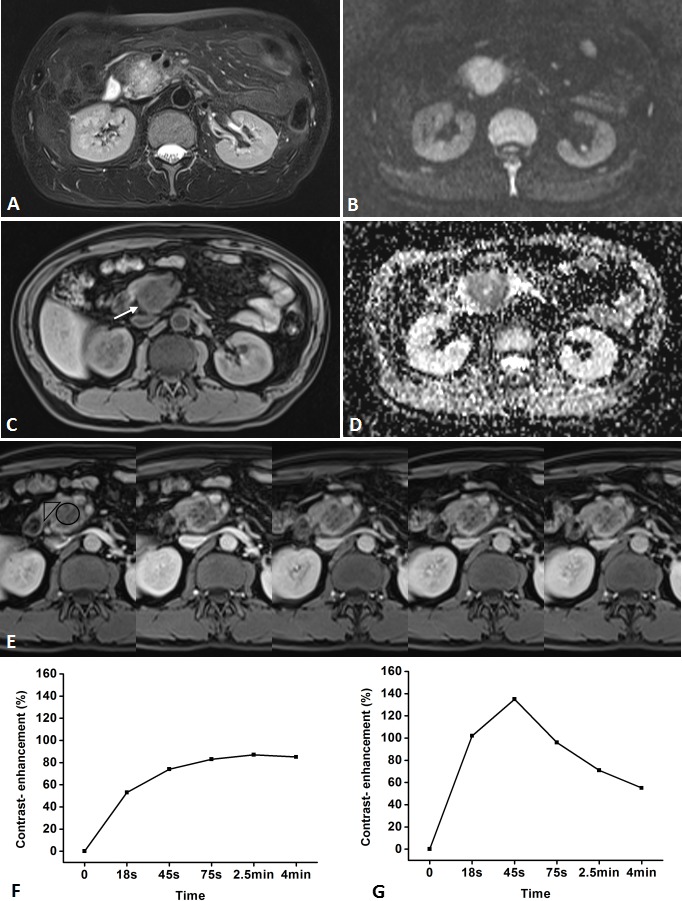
Representative pancreatic T2-weighted image (A), DWI with a b value of 600 s/mm^2^ (B), T1-weighted image (C), ADC map (D), DCE-MR images (E), and TIC profiles (F, G) in a 49-year-old man with pancreatic carcinoma in the head of pancreas (white arrow) DCE-MR images: 18s, 45s, 75s, 2.5 and 4 min aftercontrast injection with constant gray scale. The ROIs of mass and non-mass adjacent parenchyma (NAP) indicated with black circle and black triangle. Pancreatic mass demonstrates type-IV b TIC which shows a slow, gradually increasing enhancement pattern followed by a plateau, while NAP demonstrates type-II aTIC which shows a rapidly increasing then gradually decreasing enhancement pattern. DWI shows pancreatic mass is clearly seen as hyperintense with a well-defined margin.
